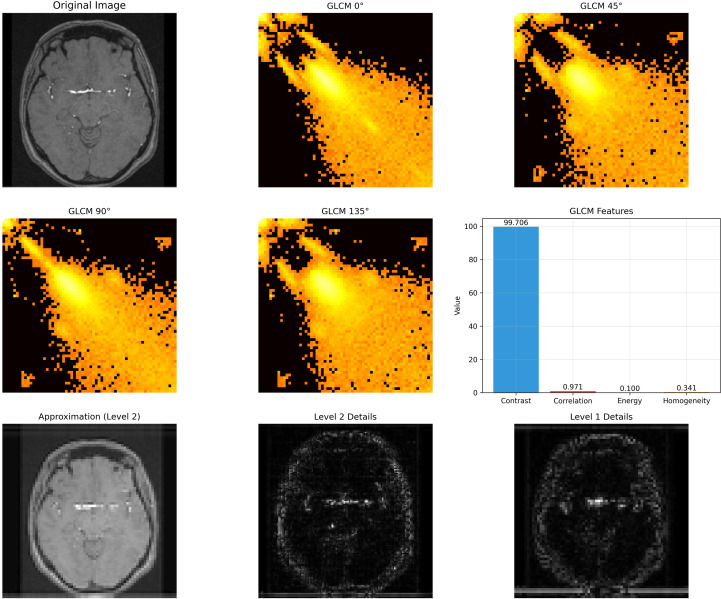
Feature extraction process from brain MRI images using GLCM and wavelet transform.
